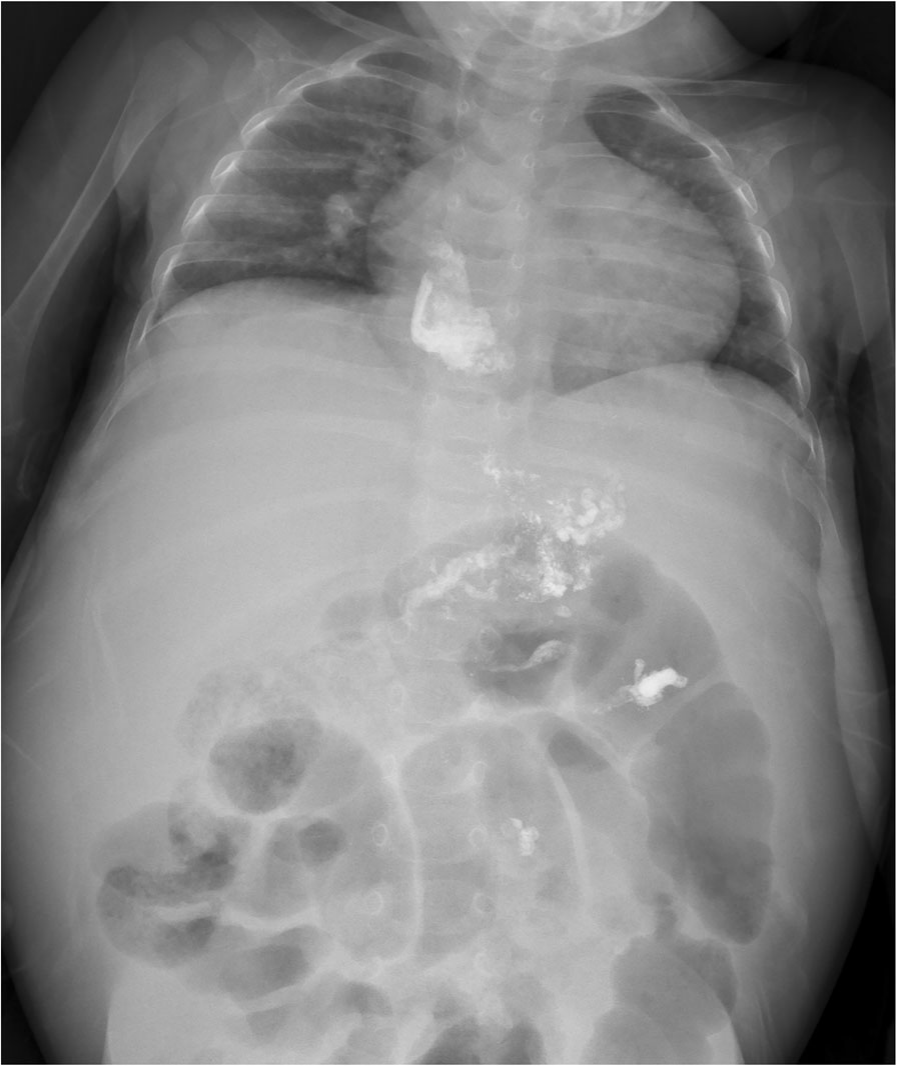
Plant X-ray film showing that the sclerosing agent was occluded in the esophageal and gastric varices and extended into the splenic vein and part of the superior mesenteric veins
